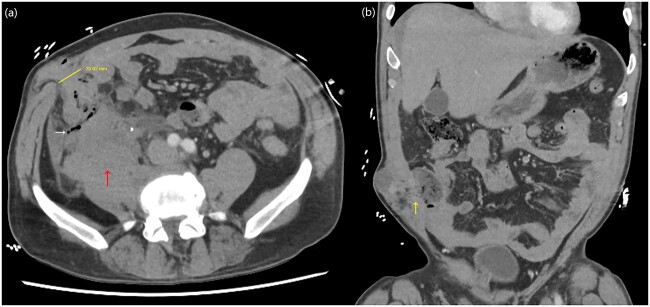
Abdominal CT axial (a) and coronal slices (b) demonstrating a 25 mm defect in the anterior abdominal wall lateral to rectus abdominis in the right lower quadrant containing caecum with adjacent small locules of extraluminal gas as well as a right-sided retroperitoneal haematoma.
